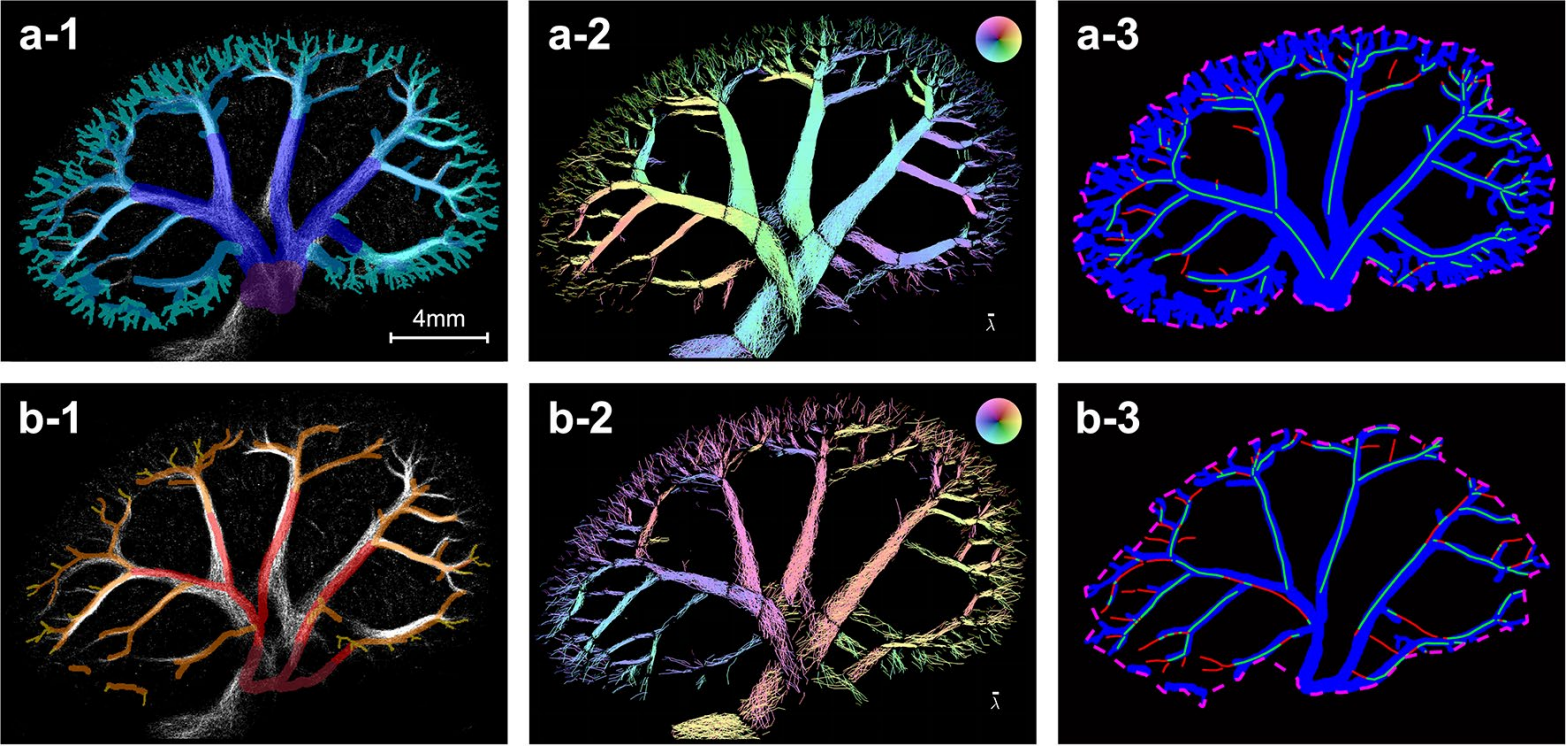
Vessel overlap estimation. (a) Veins, (b) Arteries. (a-1,b-1) Manually drawn veins and arteries on the *μ*CT in the super-resolution ultrasound scan 2 overlap (the colored *μ*CT segmentation is displayed on top of a white super-resolution ultrasound image). Purple = renal vein branches, dark blue = segmental veins, blue = arcuate veins, turquoise = cortical radial veins, dark red = renal artery branches, red = segmental arteries, orange = arcuate arteries, yellow = cortical radial arteries. (a-2,b-2) Filtered microbubble track maps including only regions labeled as “segmental or larger arcuate vessels” or “cortex” and separated into a map with only vein (a-2) and only artery (b-2) tracks, respectively. (a-3,b-3) Overlap of the manually drawn vessel centerlines in the super-resolution image (green and red lines) and *μ*CT vessel ROIs (blue) inside the *μ*CT ROI region (pink dashed line). The green centerlines are overlapping the *μ*CT ROIs while the red centerlines are not.
